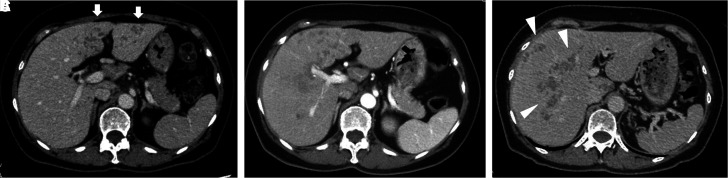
Contrast-enhanced computed tomography of the abdomen. (A) Low-density areas are visible in the liver (white arrows). (B) The previous lesions disappeared. (C) New multilocular low-density areas are seen (white arrows).
